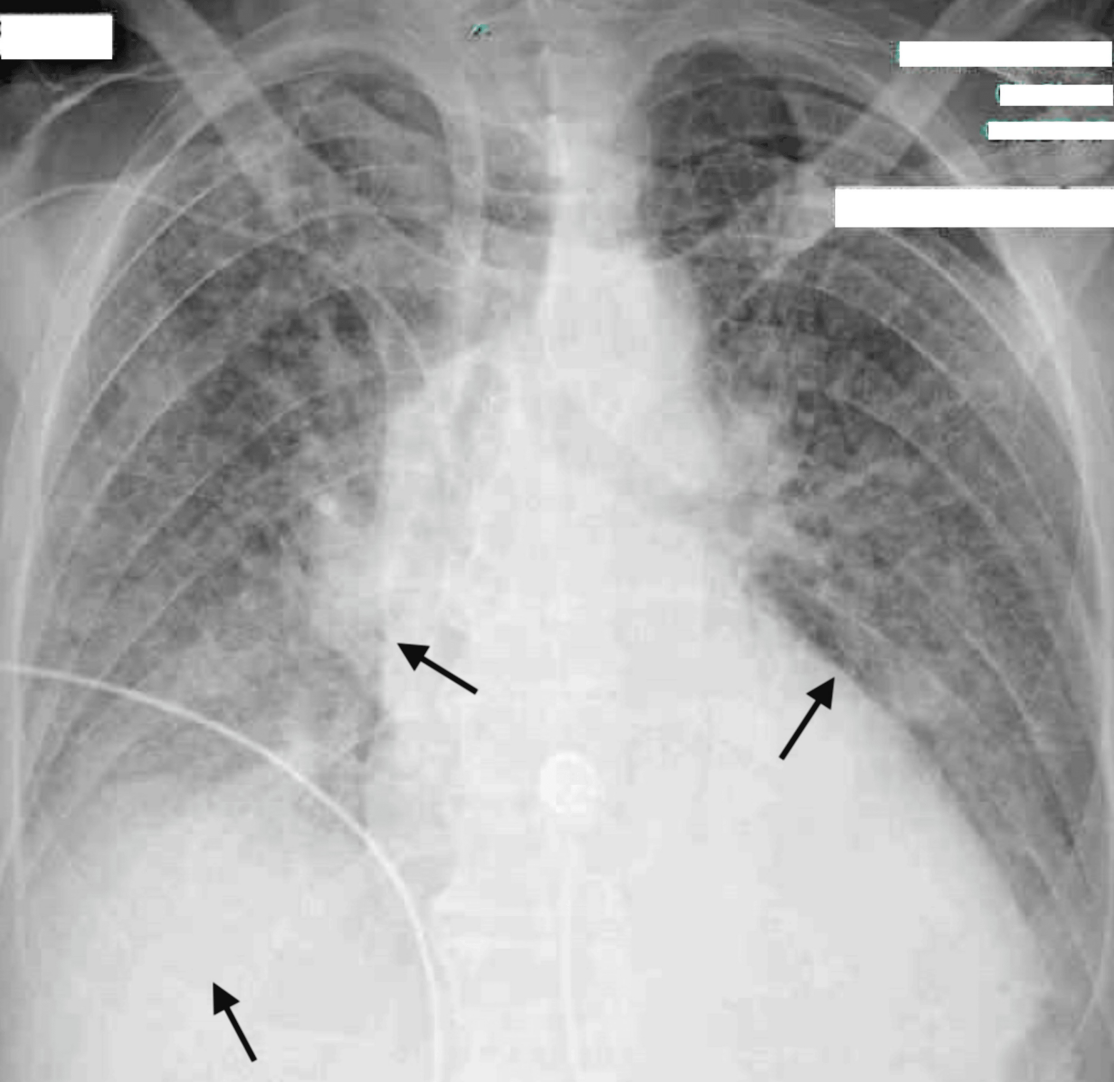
Pre-treatment CXR AP view CXR AP taken before administration of pulse dose steroids. Arrows pointing to the haziness indicate edema. CXR: chest X-ray, AP: anteroposterior
